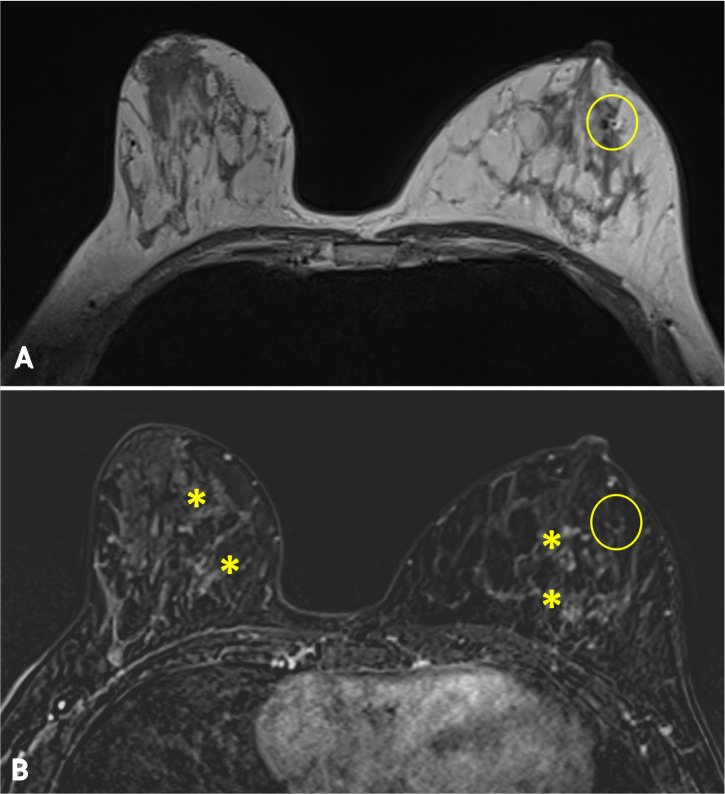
(A) Breast MRI after neoadjuvant chemotherapy. (A) Hydrophilic clip marker (circle) without (B) associated enhancement, compatible with complete response. Notice physiological parenchymal enhancement in both breasts (asterisks).
